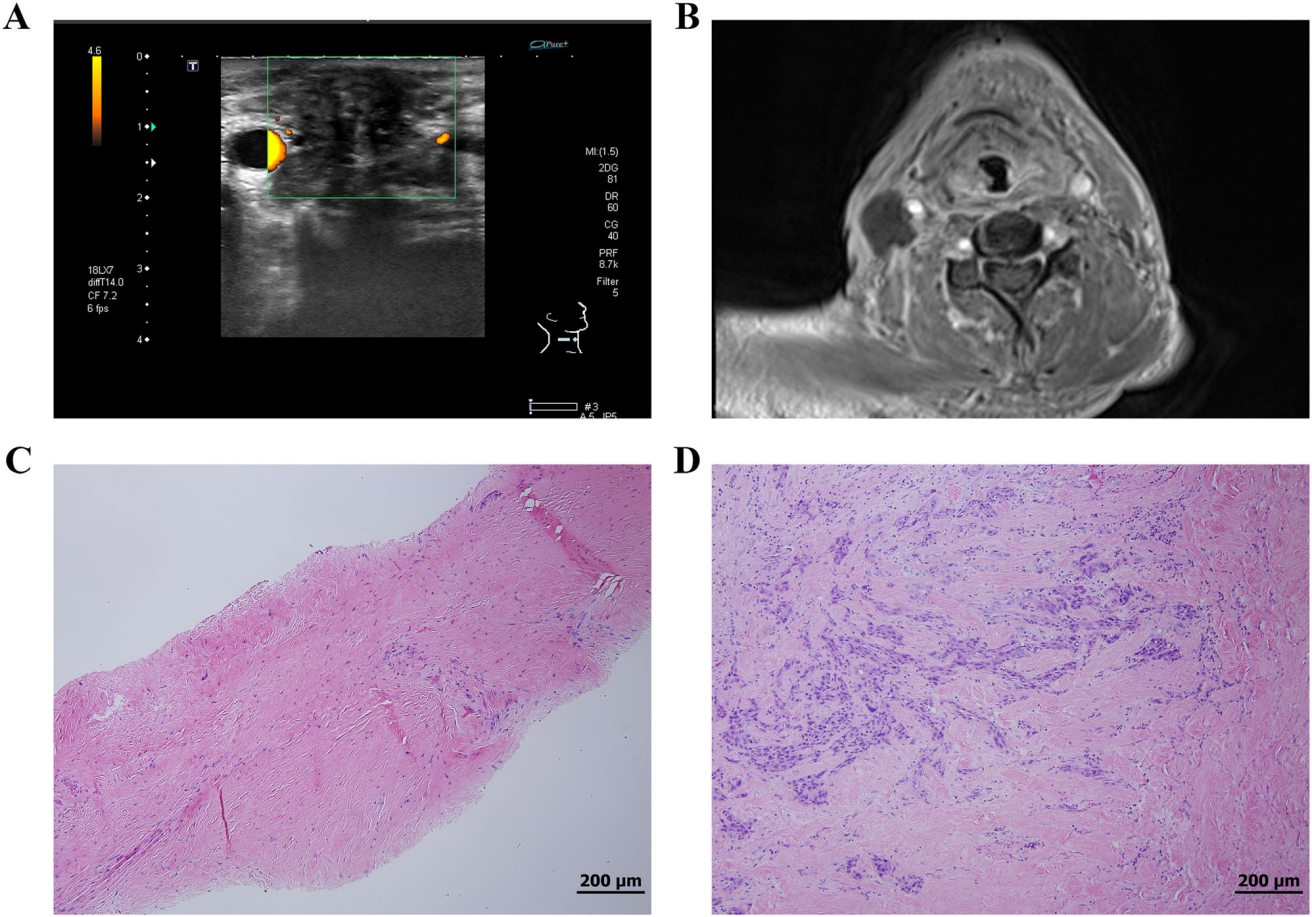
(A) Ultrasound picture of treated right tongue cancer with ipsilateral nodal mass; (B) corresponding magnetic resonance imaging showing axial view of T1 with gadolinium contrast medium; (C) core-needle biopsy revealed fibrotic tissue only; (D) Open biopsy revealed fibrotic tissue with metastatic squamous cell carcinoma and focal extracellular keratin deposition.
